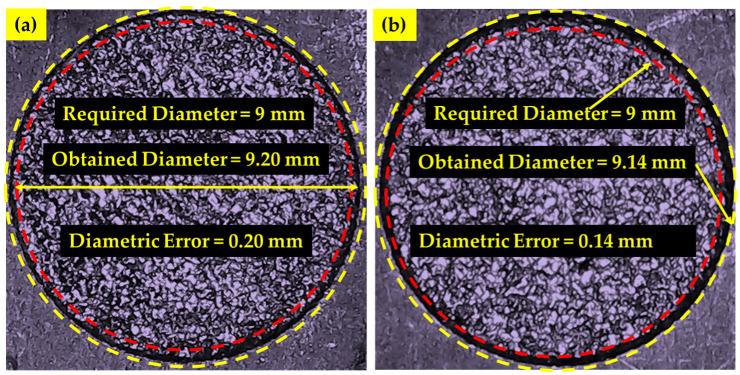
Diametric errors of machined surfaces in kerosene oil dielectric using; (a) NT electrodes; (b) CT electrodes.
